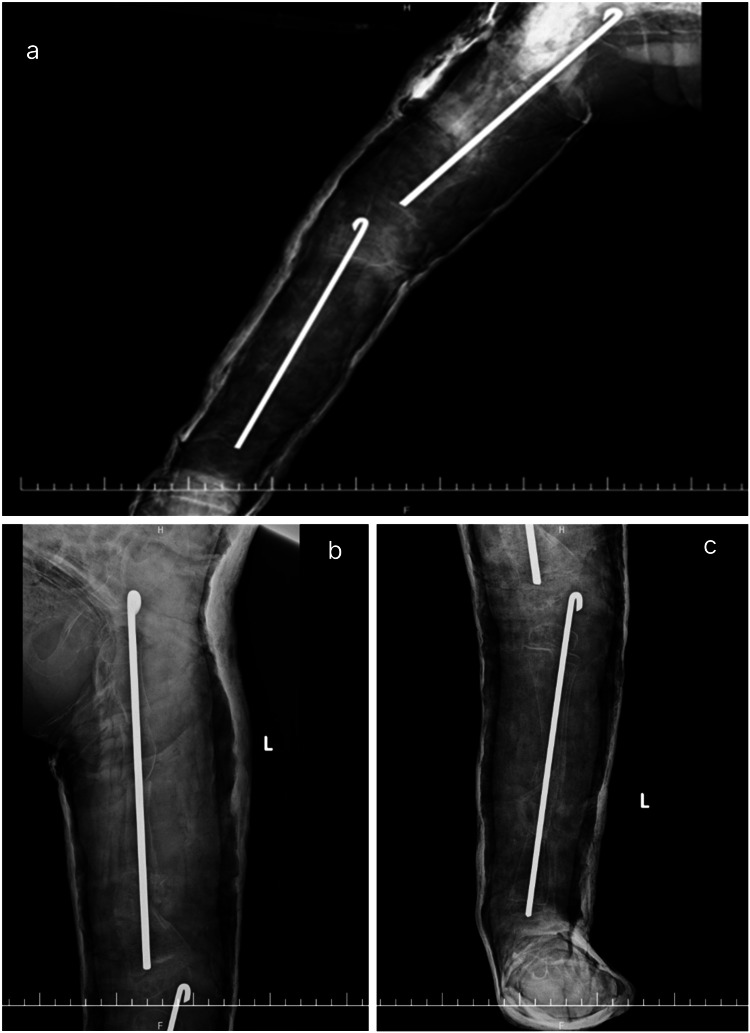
Postoperative radiograph after deformity correction of the right and left lower limbs
